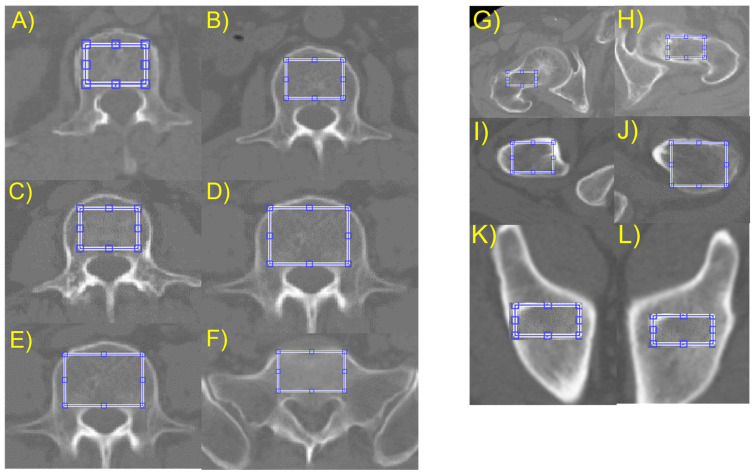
Regions of Interest in axial cuts of abdomen (left) and pelvis (right) CT. (A) Lumbar vertebra 1, (B) lumbar vertebra 2, (C) lumbar vertebra 3, (D) lumbar vertebra 4, (E) lumbar vertebra 5, (F) sacrum vertebra 1, (G) femur neck right, (H) femur neck left, (I) femur intertrochanter right, (J) femur intertrochanter left, (K) acetabulum right, and (L) acetabulum left.
